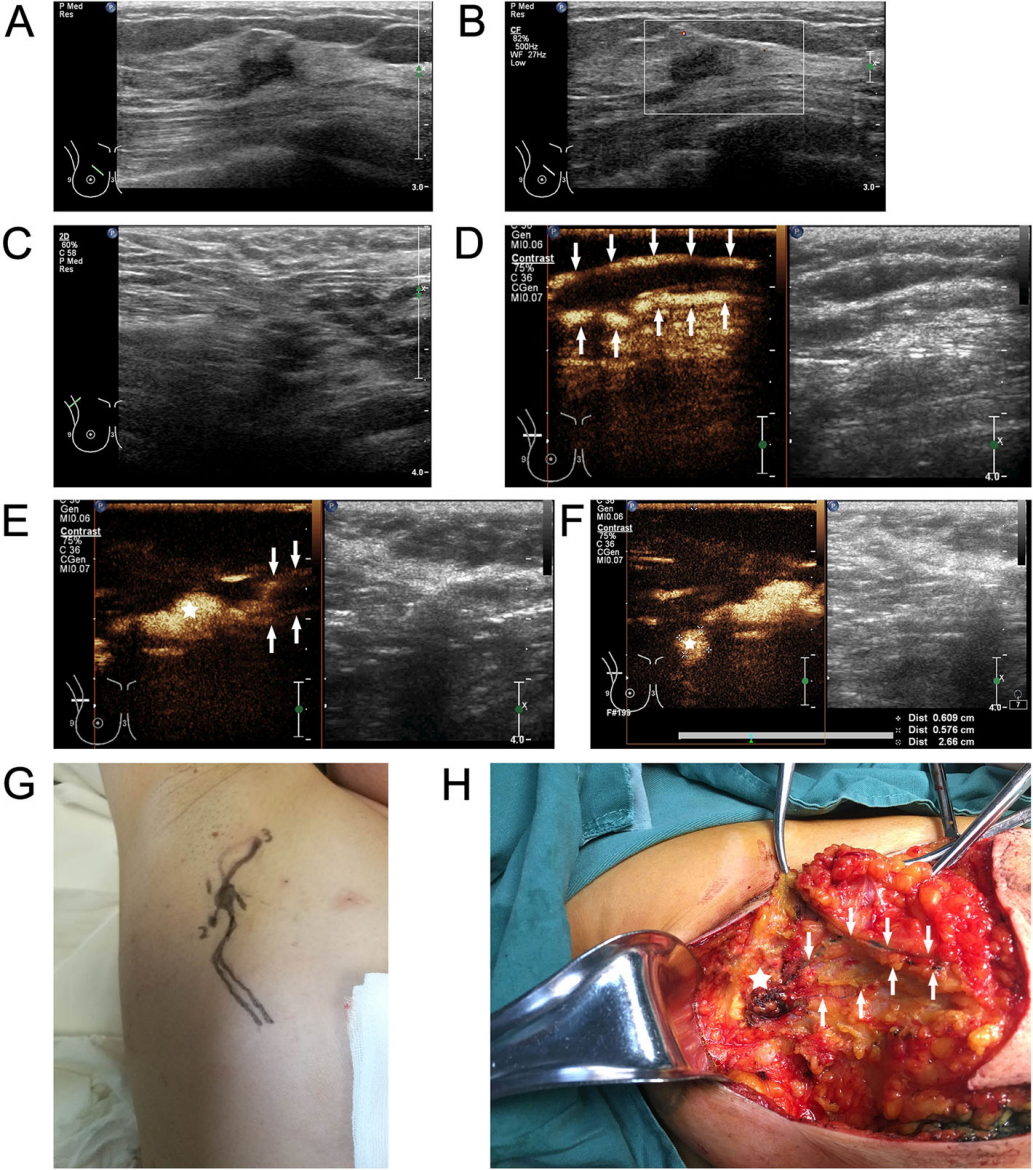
Case2, 52 year-old woman, Invasive ductal carcinoma.a B-Mode Ultrasonography of right inner upper quadrant invasive ductal carcinoma; b Color-Doppler Ultrasonography of right inner upper quadrant invasive ductal carcinoma; c B-Mode Ultrasonography of the right axillary; dTraced two enhanced lymphatic vessel (arrows) after injecting the UCA; e Both lymphatic vessel (arrows) drain to the same enhanced SLN (asterisk); fUCA can continue to drain to subsequent enhanced lymph node (asterisk); g The body surface projection of enhanced lymphatic vessel and lymph node; hTraced two dyed lymphatic vessel (arrows) drain to the same enhanced SLN (asterisk) after injecting the MB before surgery, the lymphatic drainage pattern traced by CEUS was consistent with MB
